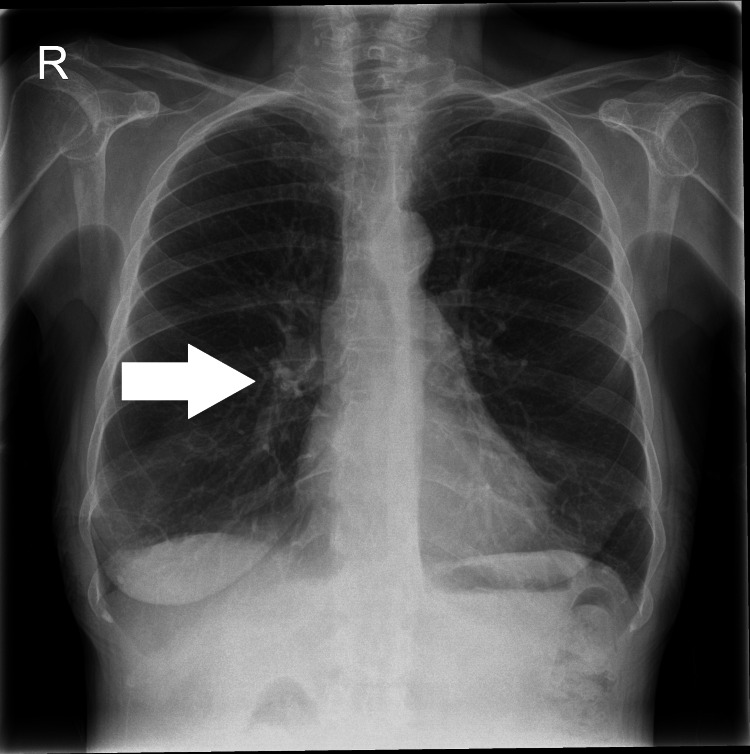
Chest Radiograph Highlighting Bronchovascular Markings Posteroanterior chest radiograph demonstrating prominent bronchovascular markings (white arrow), consistent with chronic airway changes.
